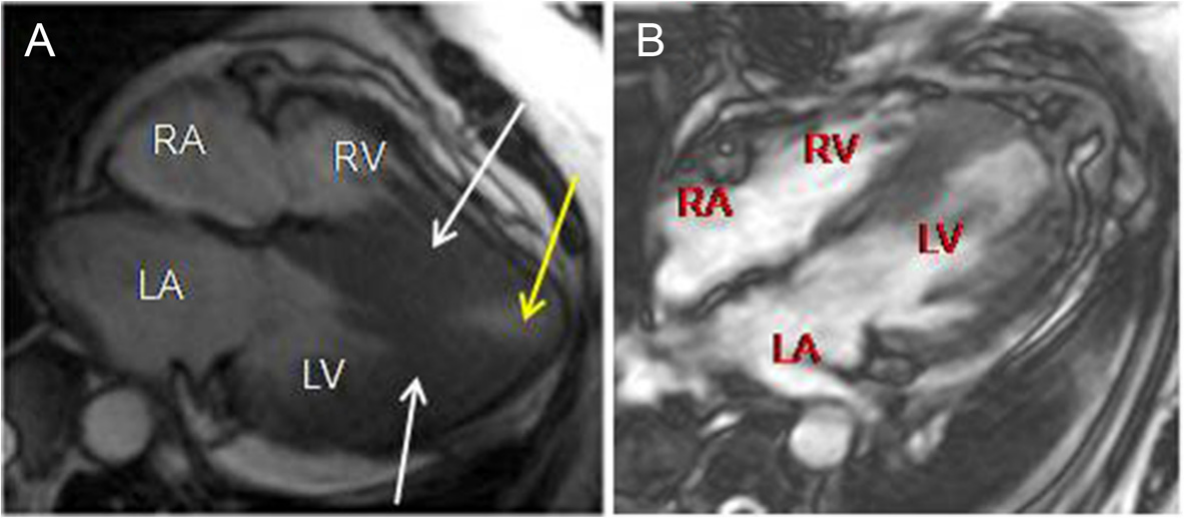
Nuclear magnetic resonance images of the patient. (A) Long-axis, four-chamber nuclear magnetic resonance (NMR) scan taken in end-systole shows hypertrophy confined to the mid-apical left ventricle (LV), causing obstruction of the LV (white arrows). Note the formation of the apical aneurysm (yellow arrow). (B) Post-surgery NMR image shows a reduction in septal thickness. LA, Left atrium; RA, Right atrium; RV, Right ventricle.
